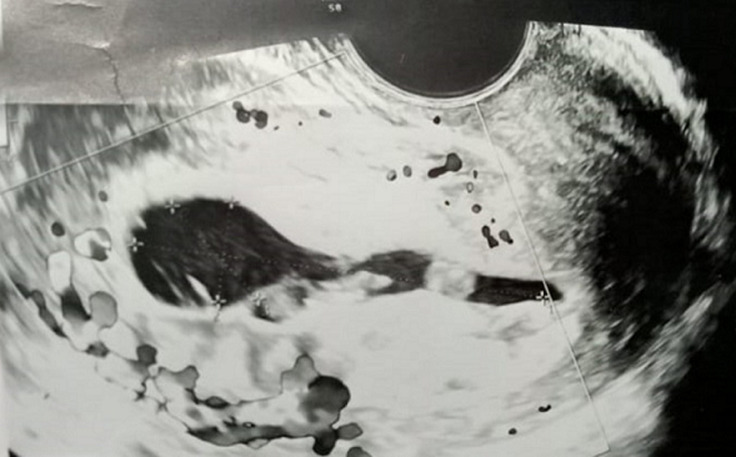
Doppler study demonstrating uterine arteriovenous malformation
